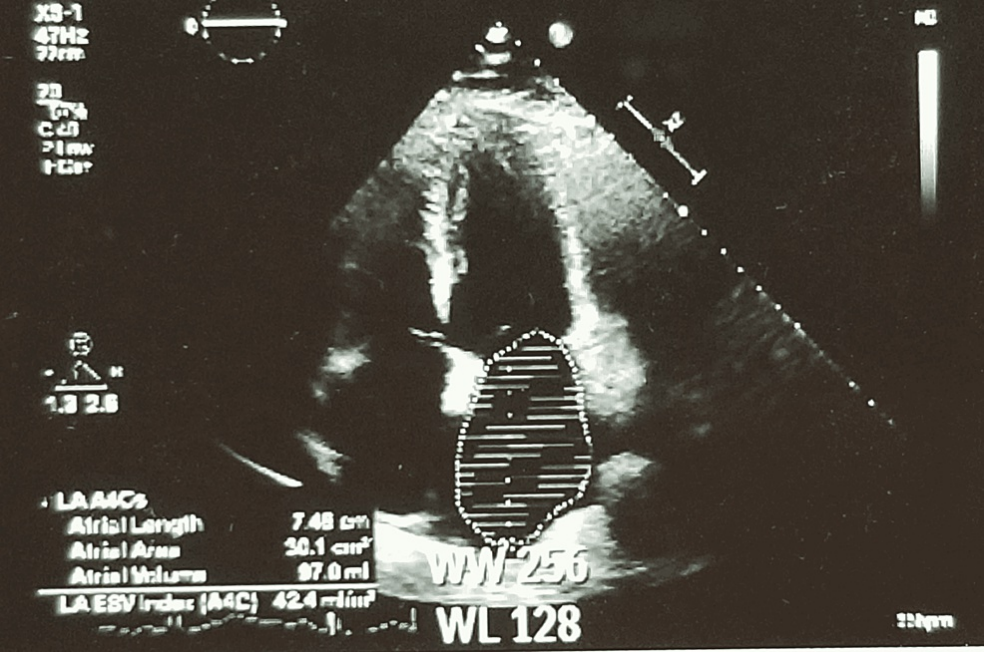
2D Echocardiogram demonstrating moderately dilated left atrium with a left atrial volume index of 42.4ml/m^2
